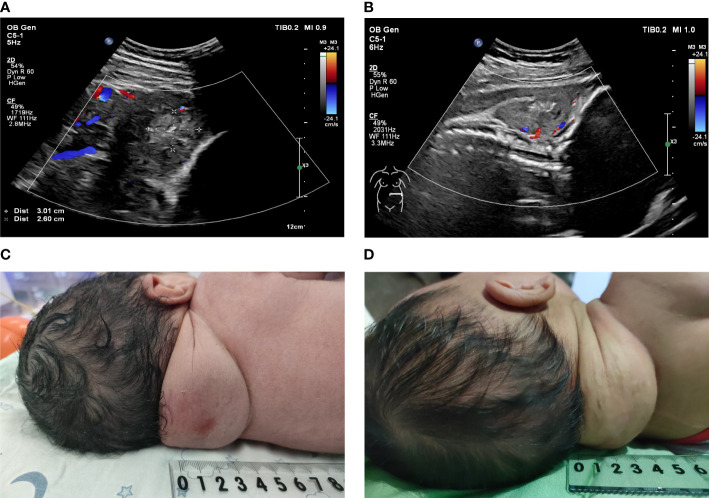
(A, B): Doppler ultrasonography was used to observe the blood flow signal of the fetal posterior neck tumor after surgery. (A) One week after surgery; (B) One month after surgery. (C): A solid and soft mass of approximately 6 × 5 × 4 cm protruded from the posterior neck of the newborn. (D): A solid and soft mass of approximately 5 × 3 × 3 cm protruded from the posterior neck of the 42-day-old infant.
